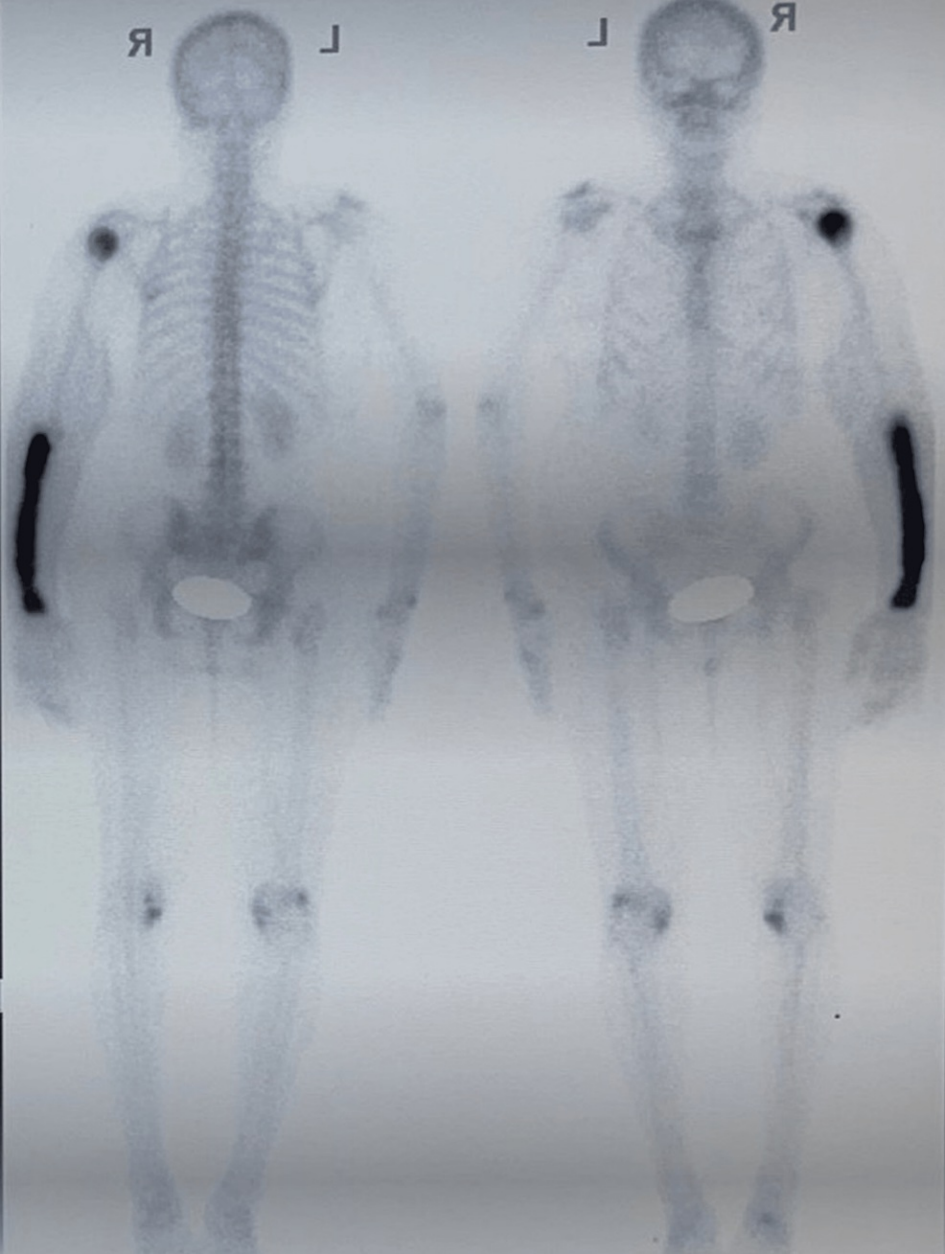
A technetium-99m bone scintigraphy study (bone scan) showing focal uniform tracer uptake in the right radius corresponding to the bony lesion as well as in the right proximal humerus suggestive of bone metastasis.
